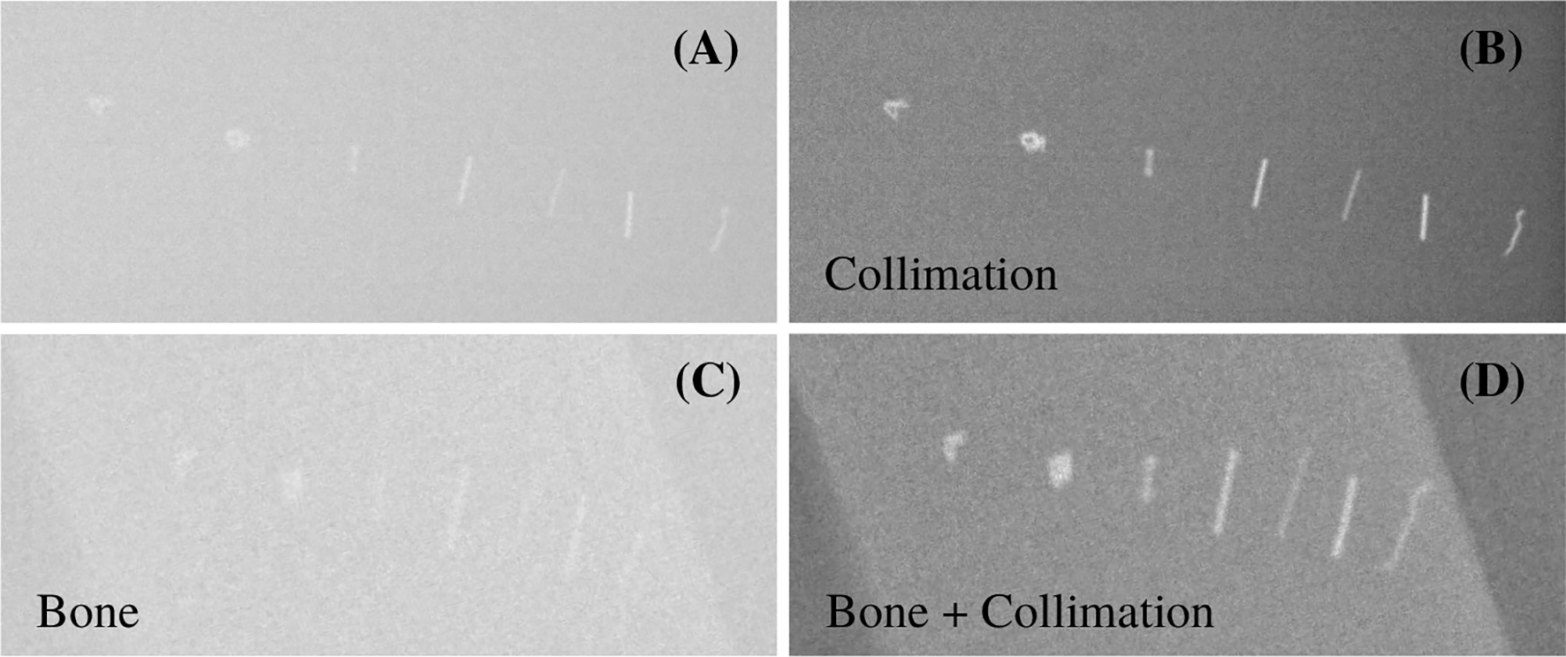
X-ray images of 7 different fiducial markers for the different settings described in Table 2. From left to right: Gold Anchor (6), Gold Anchor (7), ZrO_2_ (8), Viscoil (2), Visicoil (3), Visicoil (4), and Visicoil (1), listed in Table 1. (A, B) show the images without and with collimation. (C, D) show the images with the bone slabs in front of the markers without and with collimation, respectively.
